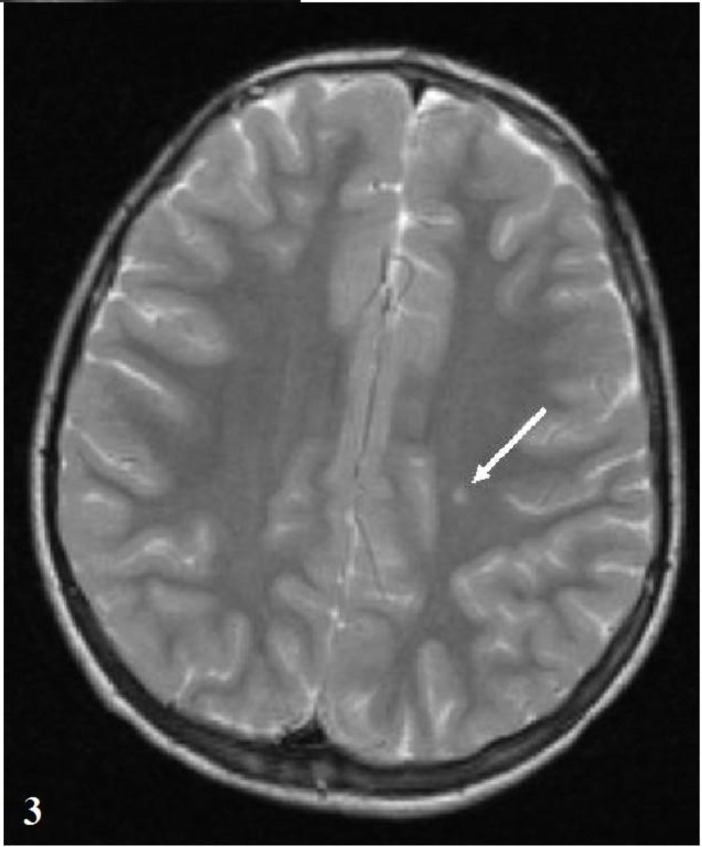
T2-weighted axial image shows a single hyperintense focus in the left frontal white matter (Patient 5).
